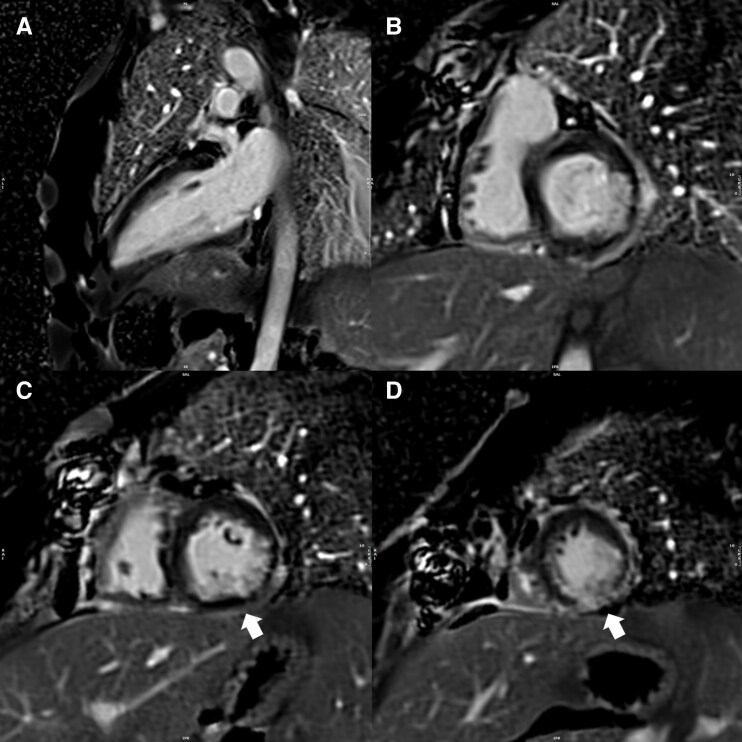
CMR. Cardiac MRI revealing ischaemic scarring. (*A*) Two-chamber view demonstrating subendocardial LGE with 75% of transmurality in the inferior wall. (*B–D*) Basal to apical SAX view also highlighting LGE in the inferolateral and inferior walls (arrows). SAX, short-axis; LGE, late gadolinium enhancement.
